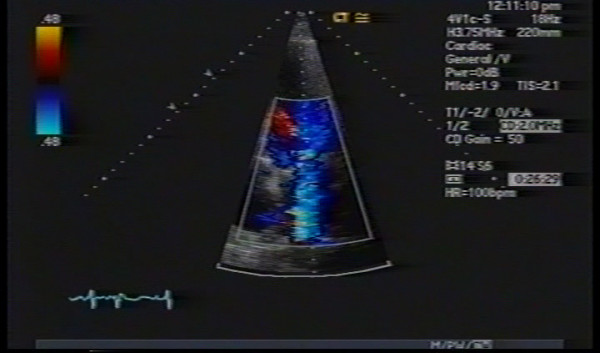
Transthoracic Echocardiogram showing (arrow) possible mitral valve regurgitation.
